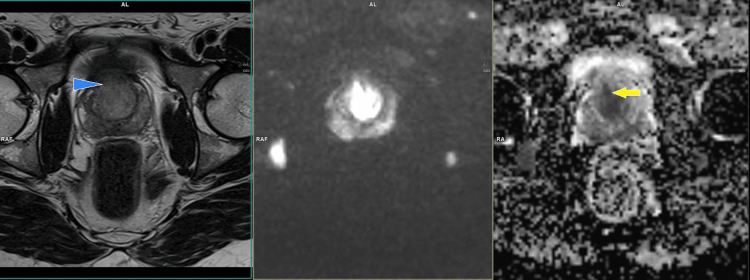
Biparametric prostate MRI of a 50-year-old patient showing diffuse tumor infiltration of the whole gland with the index lesion in the anterior stroma zone, which shows strong restriction in the diffusion sequence. The lesions appear hypointense in the axial T2 sequence (whole arrow) and the axial ADC map (arrowhead).
